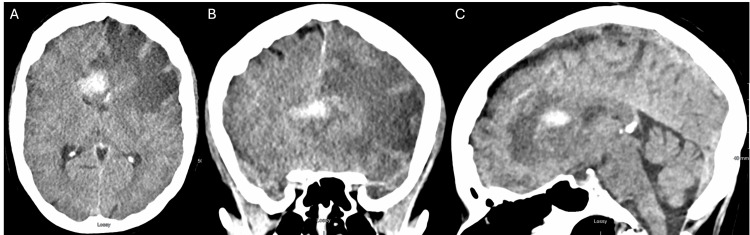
A non-contrast brain CT scan. Axial (A) and coronal (B) views show marked perilesional edema causing a left-ward midline shift. The hemorrhage is located between the body and the genu of the corpus callosum (C).
